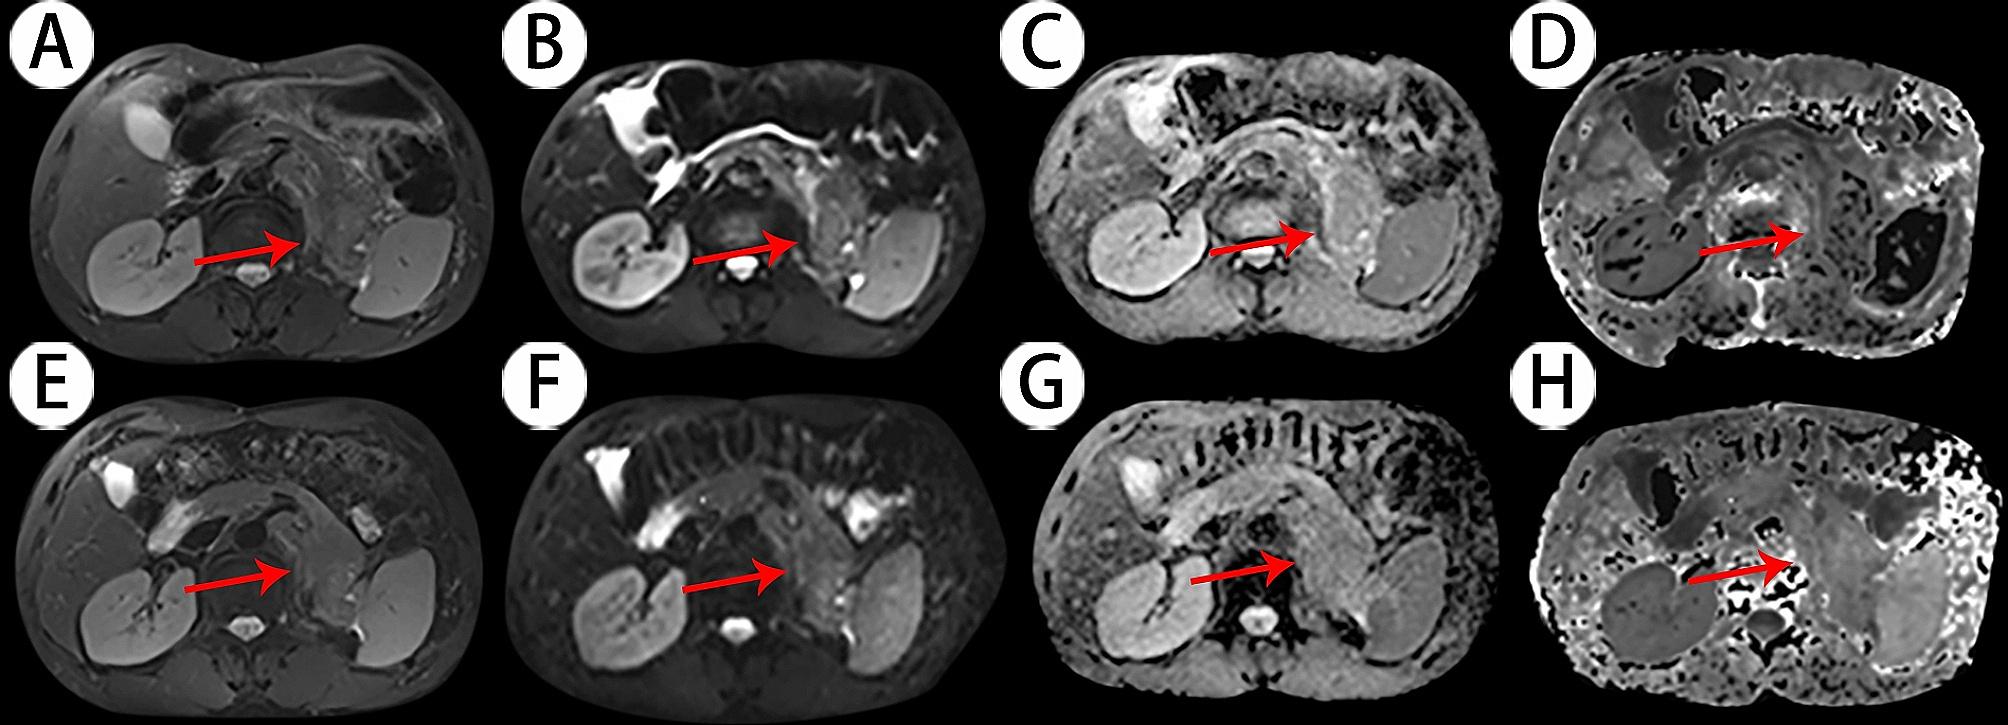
A and E: T2-weighted images; B and F: Diffusion weighted image s(DWI) with b-value 0 s/mm^2^; C and G: Apparent diffusion coefficient (ADC) maps; D and H: Diffusion kurtosis imaging (DKI) maps. Images A to D represent the lesion before chemotherapy, while images E to H represent the lesion after 2 courses of chemotherapy. Red arrow: the pancreatic lesion. The ADC and MD values increased slightly, and the K value increased slightly after two courses of chemotherapy. The mass increased slightly after two courses of chemotherapy and was evaluated as non-PR. This patient is a 58-year-old male patient with a 4.8 × 3.5 cm mass in the tail of the pancreas accompanied by liver metastasis. Here are the related parameters: ADC_pre_=1.02 × 10^− 3^ mm^2^/s, ADC_post_=1.12 × 10^− 3^ mm^2^/s, ADCchange = 0.10; MK_pre_=0.64, MK_post_=0.65, MK_change_=0.01; MD_pre_=1.83 × 10^− 3^ mm^2^/s, MD_post_=2.07 × 10^− 3^ mm^2^/s, MD_change_=0.13
